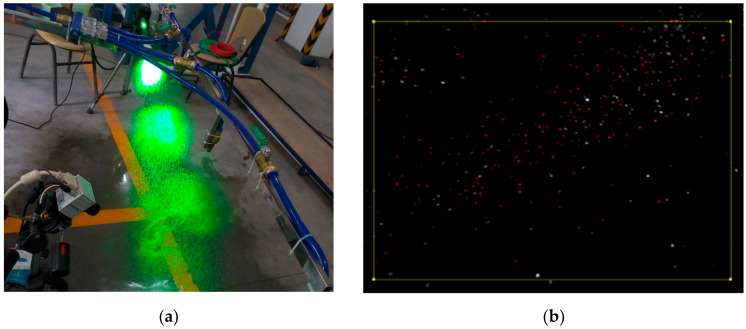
Doppler particle sensor experiment. (a) Doppler particle sensor measuring droplet size; (b) Doppler particle sensor capturing particle image.
